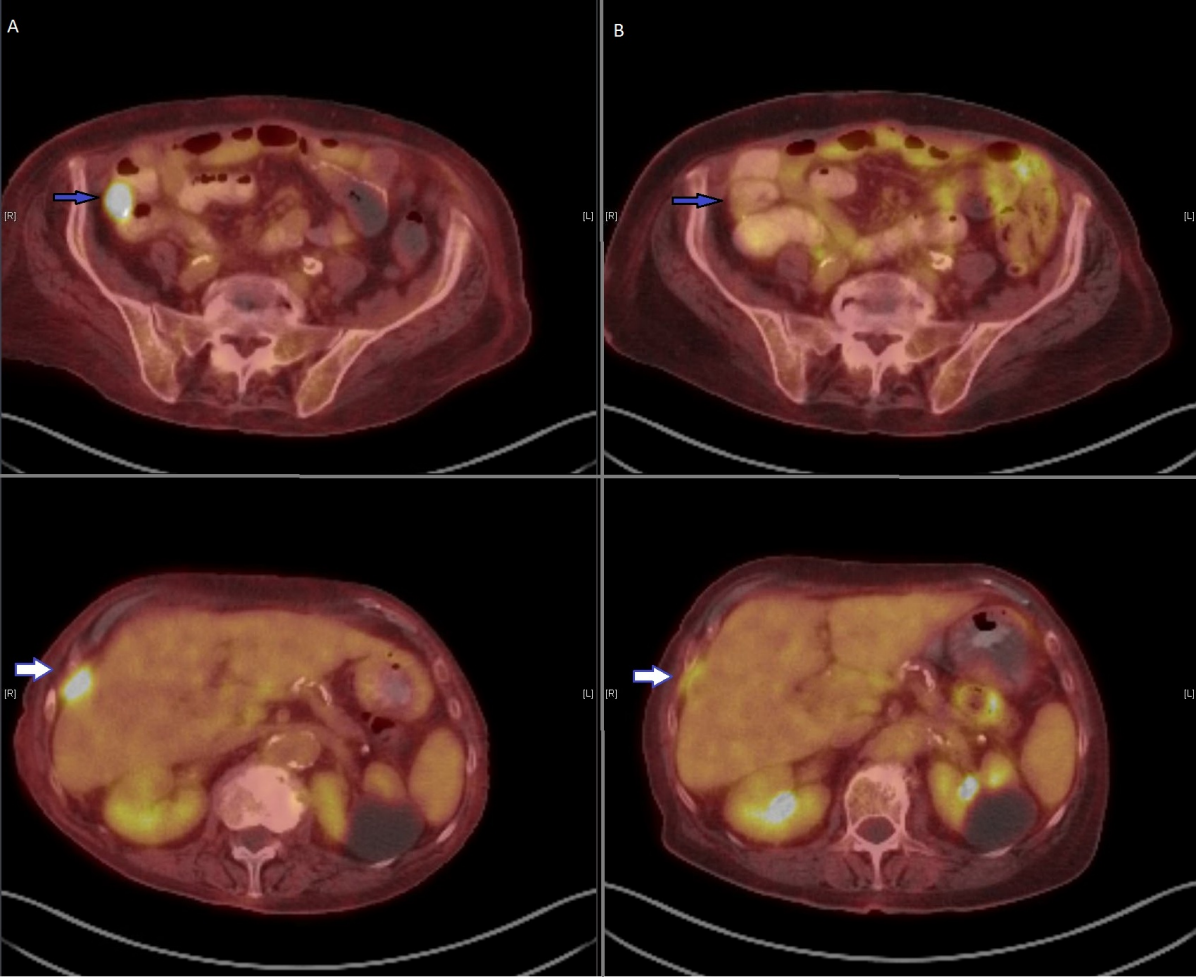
Response before and after regorafenib measured by PET/CT scan PET scan before (left panel A) and three months after low dose regorafenib (right panel B), showing improvement in right lower quadrant anastomotic soft tissue mass (blue arrow), and liver metastasis (white arrow)
